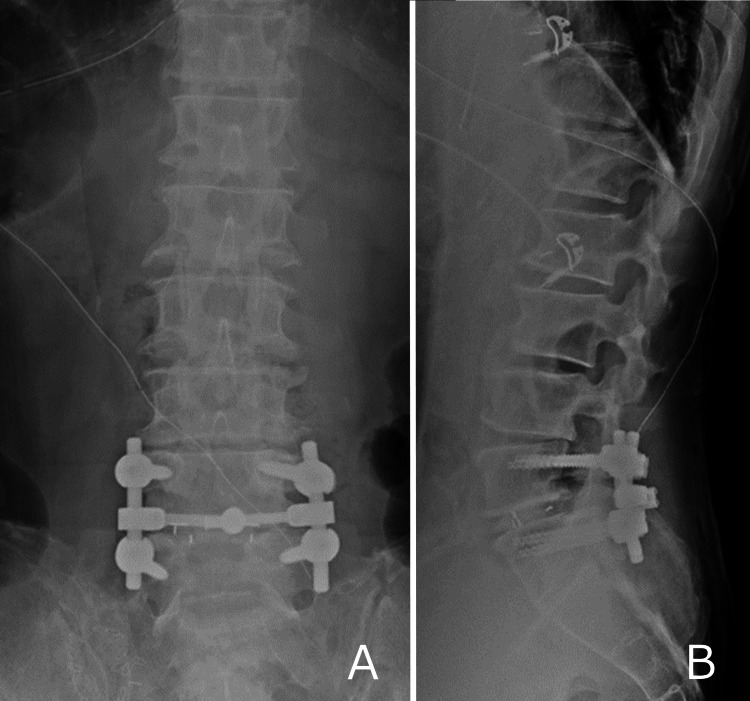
Postoperative X-ray images After L5/S1 transforaminal lumbar interbody fusion. (A) Anteroposterior view. (B) Lateral view.
